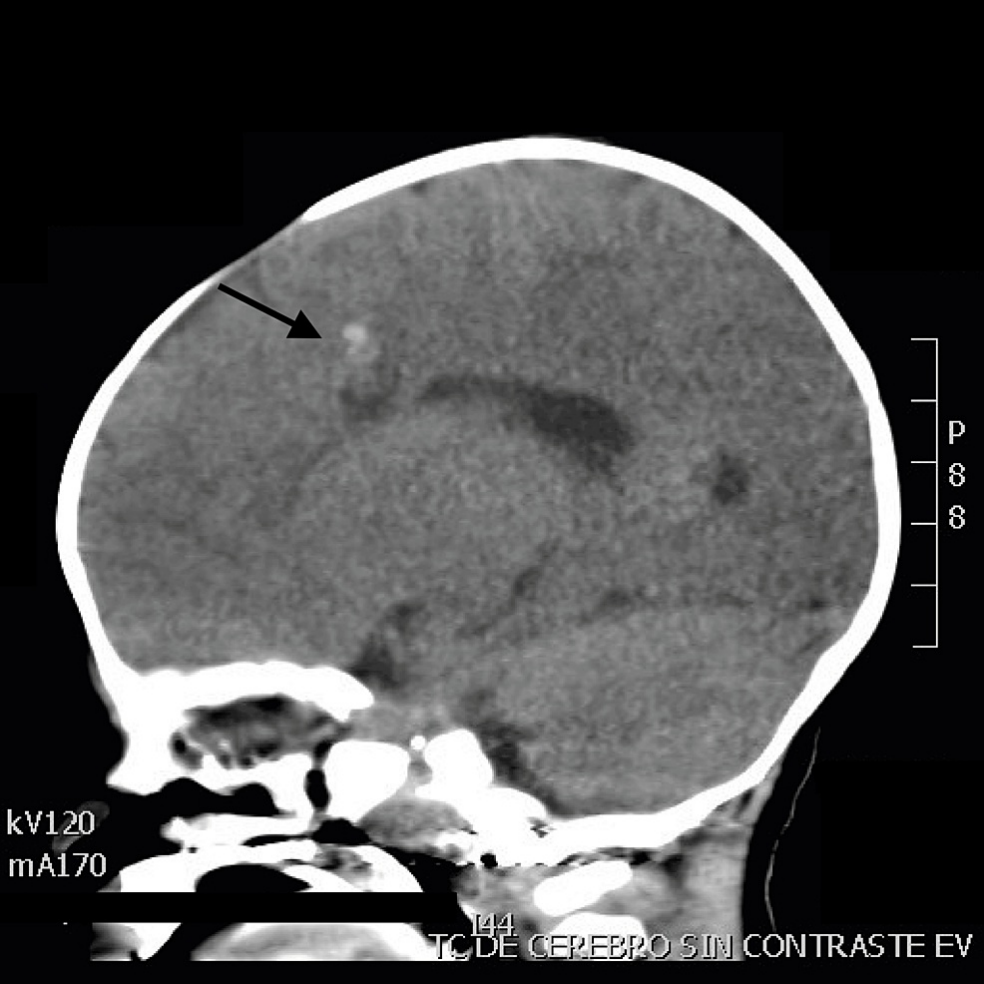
Sagittal plane of CNS CT without contrast. The patient’s largest cavernous malformation is shown in the roof of the left ventricle (black arrows). CNS, central nervous system; CT, computed tomography
